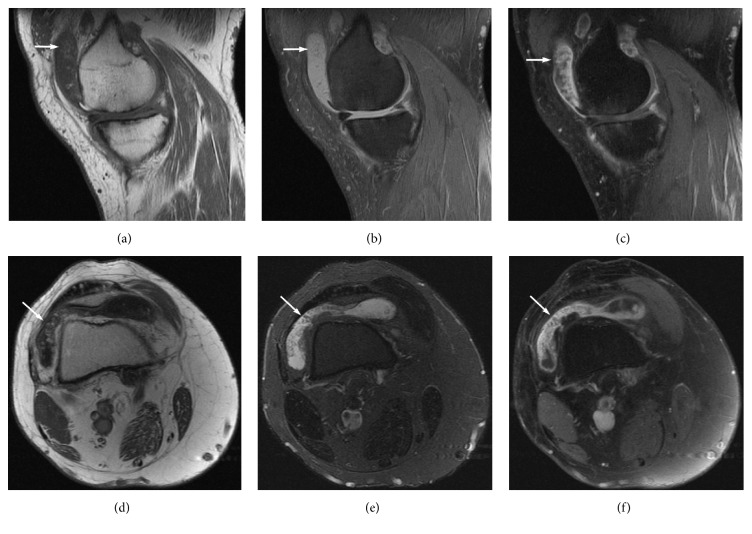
MR images at the same sagittal level on T1-weighted (a), PD-weighted with FS (b), and T1-weighted with FS after iv administration of gadolinium (c) demonstrate medial compartment osteoarthritis with marginal osteophytes, chondral lesions, subchondral changes, and a complex tear of the posterior horn of medial meniscus. Mild villous frond-like fatty subsynovial hyperplasia is present in the suprapatellar bursa associated with synovial proliferation, which is enhanced after iv administration of gadolinium, and joint effusion (arrows). MR images at the same axial level on T1-weighted (d), PD-weighted with FS (e), and T1-weighted with FS after iv administration of gadolinium (f) confirm the above findings (arrows), which are strongly suggestive of LA.
